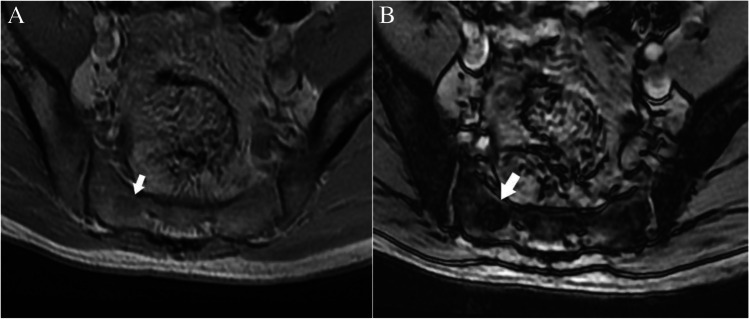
Chemical shift imaging. 56-year-old man with incidental lesion in the right sacral ala. The lesion (arrows) is isointense to skeletal muscle on the A in-phase T1-weighted gradient echo MR image and has uniform loss (drop) in signal on the B oppose phase T1-weighted gradient echo MR image indicating the presence of internal fat. The lesion is compatible with a focus of red marrow
